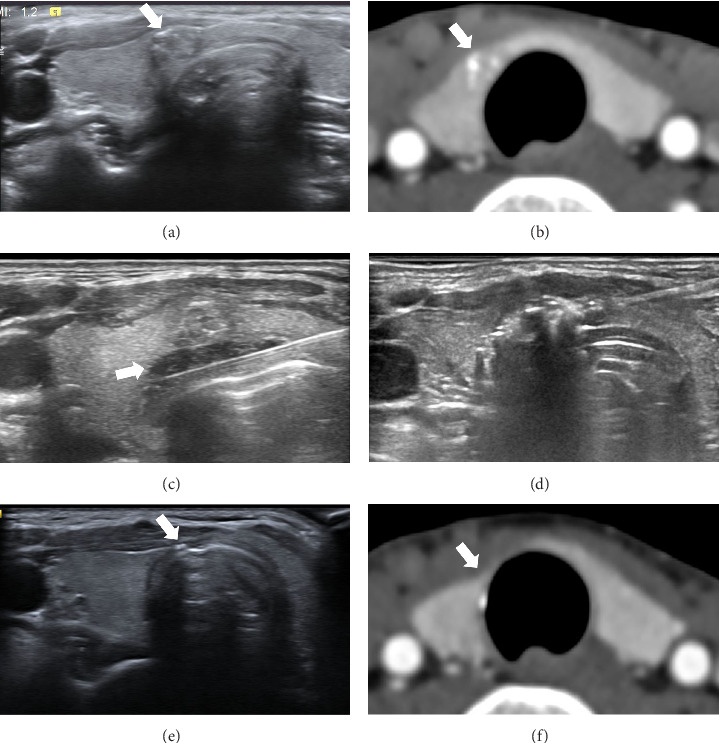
A 46-year-old Taiwanese female with PTMC who underwent RFA. (a) US examination and (b) contrast enhanced CT scan revealed the PTMC (arrows) measuring 7 × 6 × 4 mm in the right isthmus of the thyroid adjacent to the trachea. (c) In the beginning of the RFA procedure, a layer of hydrodissection (arrow) was created to prevent thermal injury to the adjacent trachea. (d) During the RFA procedure, the targeted area was transformed to a transient hyperechoic state. (e) Follow-up US examination at 1 year after RFA showed regression of the PTMC, with a remaining linear scar (arrow) measuring 2 mm and atrophy of the right isthmus of the thyroid. (f) Contrast enhanced CT scan 3 years after RFA showed total regression (arrow) of the PTMC. PTMC = papillary thyroid microcarcinoma, RFA = radiofrequency ablation, CT = CT, US = ultrasound.
